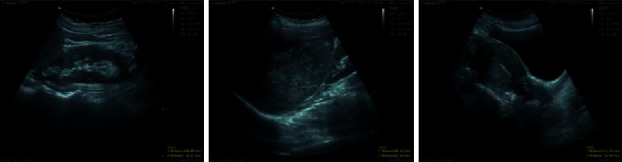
Patient's hepatobiliary ultrasound.
